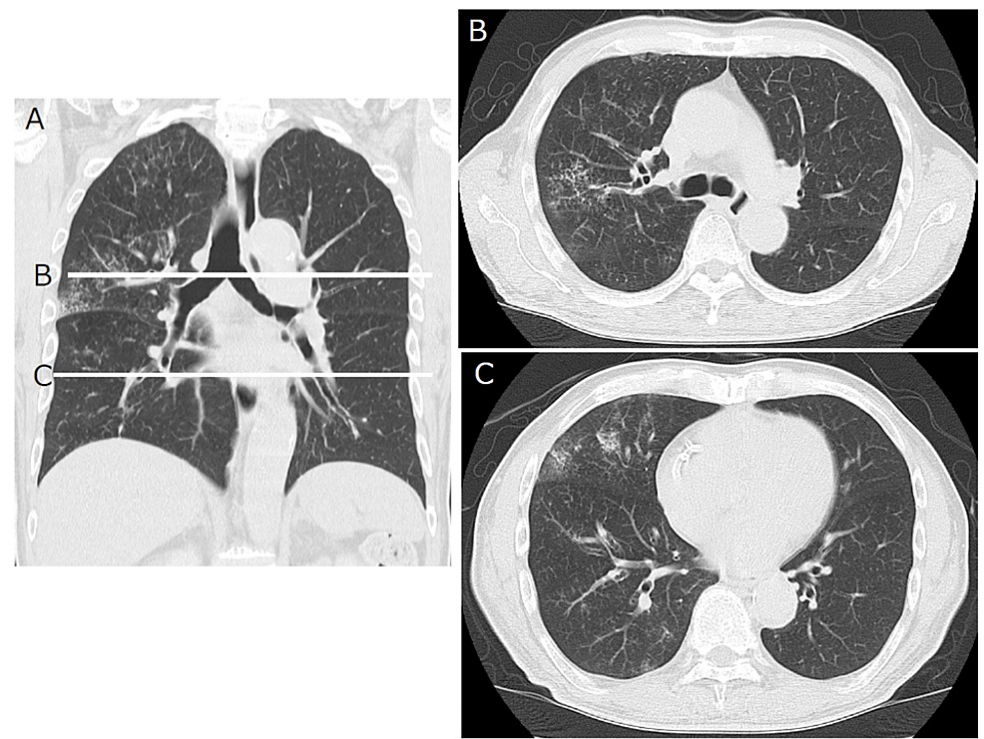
Chest CT imaging of atypical COVID-19 pneumonia. Unilateral GGO makes a score of "1" using the Fukuchi-Manabe score (A) Coronal chest CT images. (B) Tracheal bifurcation level. (C) Heart level CT: computed tomography, COVID-19: coronavirus disease 2019, GGO: ground-glass opacity
